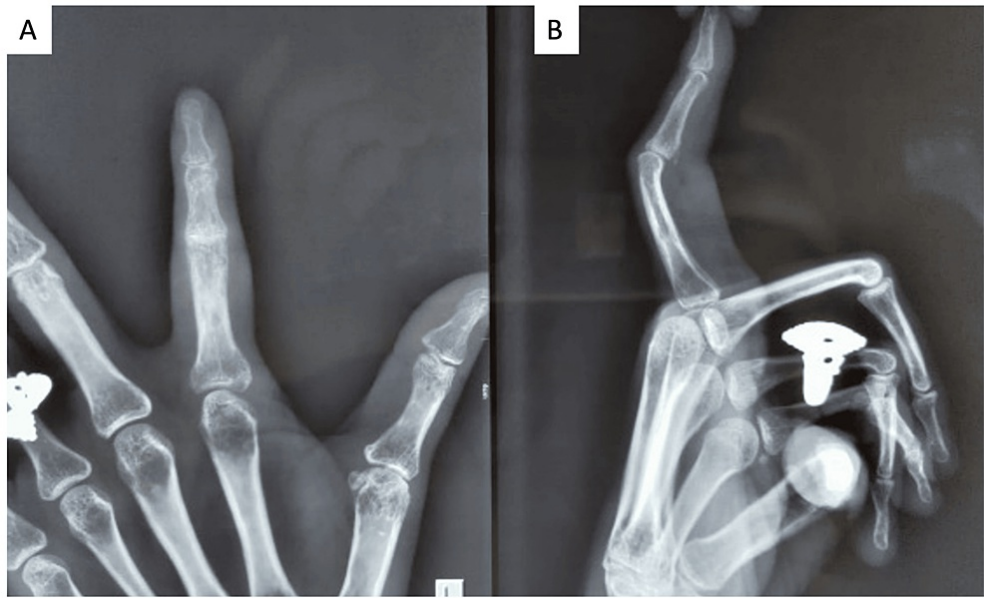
Figure A shows a one-year follow-up radiograph antero-posterior view of the hand focused on the index finger. Figure B shows the lateral view of the index finger showing no subluxation or dislocation.
